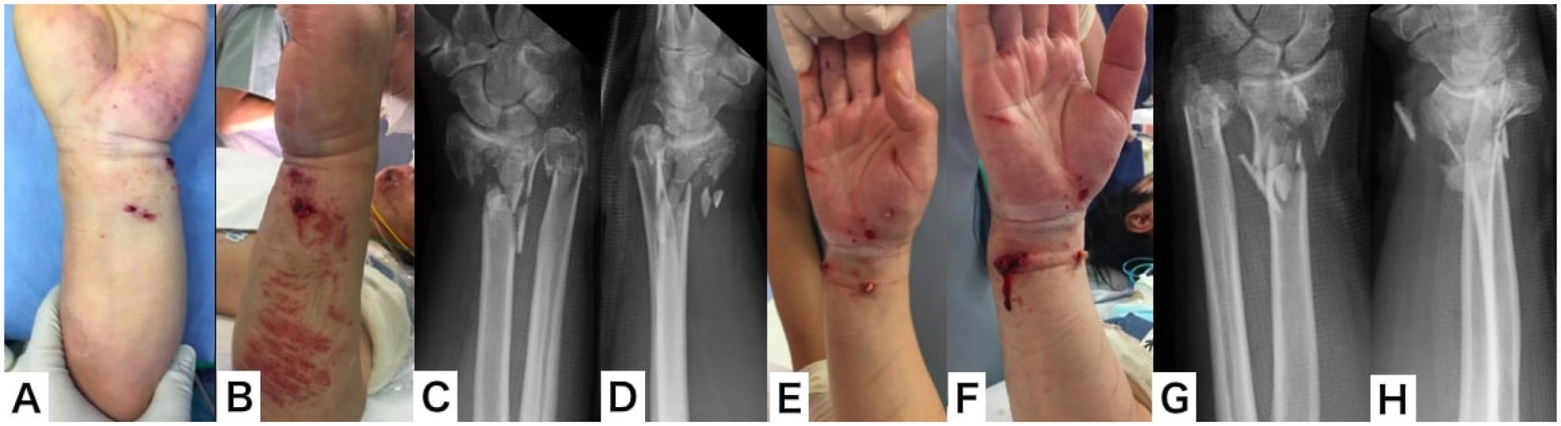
Appearance and plain x-ray on arrival at the emergency department. (A,B) Appearance of the left wrist; (C,D) plain x-ray of the left wrist; (E,F) appearance of the right wrist; (G,H) plain x-ray of the right wrist.
